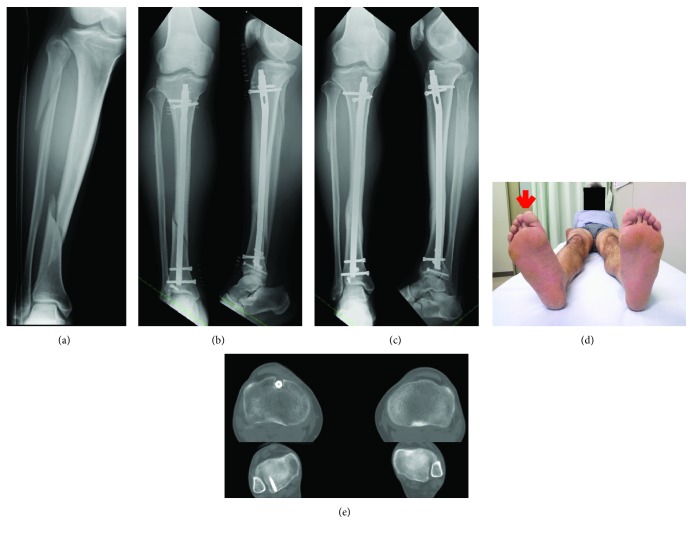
(a) Initial posttraumatic anteroposterior radiographs of the right tibia and fibula. (b-c) Anteroposterior and lateral radiographs of the right tibia obtained (b) immediate postoperatively and (c) 1 year postoperatively. (d) Photograph. (e) Computed tomography (CT) images of the right and left tibia of the patient show external tibial torsion.
